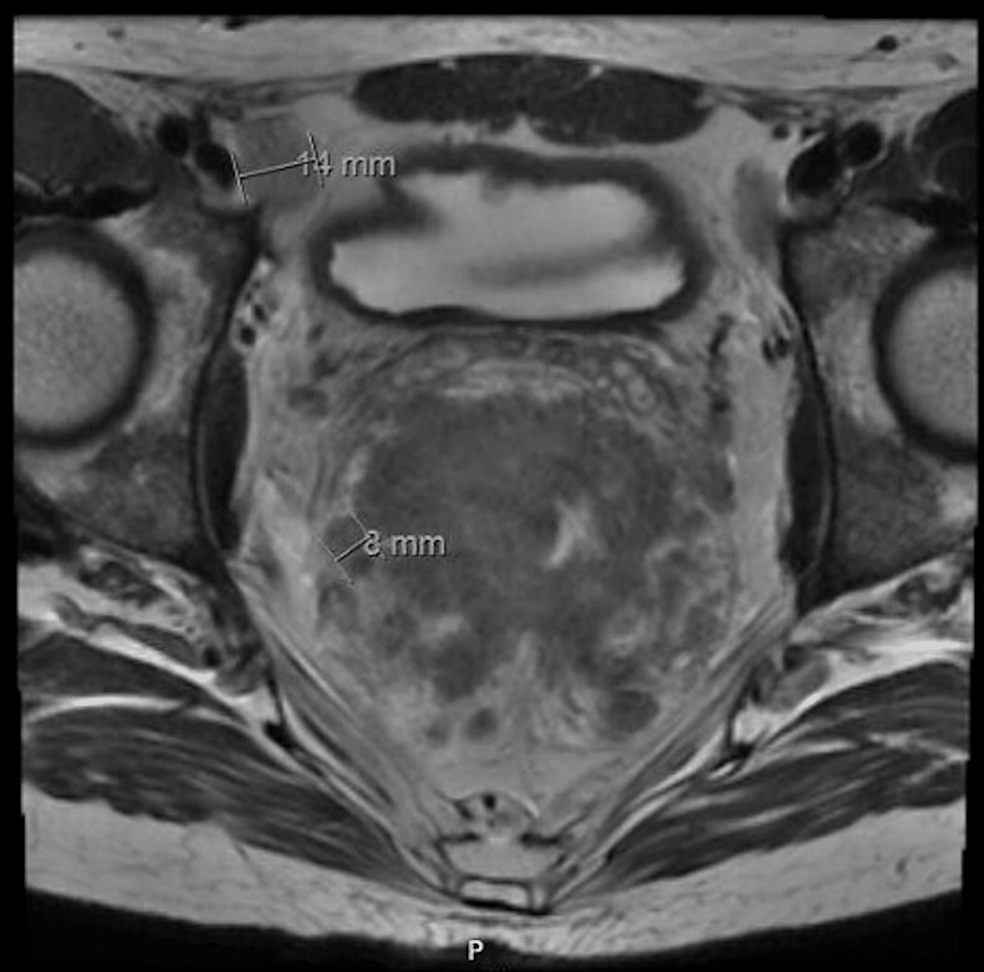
Axial thin slice, fast-recovery fast spin-echo T2-weighted MRI; measurements of two involved lymph nodes
